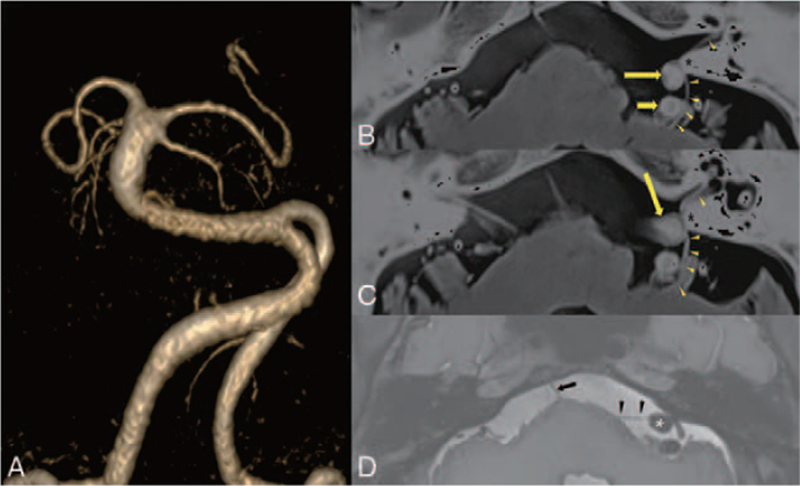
Magnetic resonance imaging (MRI) and angiography (MRA). 3D time-of-flight (TOF) MRA shows laterally displaced course of the distal segments of the intracranial vertebral arteries and mid to proximal segment basilar artery (A). Fusion image of proton density MR cisternography and TOF-MRA shows distal cisternal segment of the left facial nerve (arrowheads in B) and the left vestibulocochlear nerve (arrowheads in C) are jammed in between the laterally deviated dolichoectatic right vertebral artery (long arrow) and posterior edge of the internal acoustic meatus (asterisks). The proximal cisternal segment of the left facial nerve (arrowheads in B) is stretched by the left vertebral artery (short arrow). Axial proton density MR cisternography (D) demonstrates a laterally deviated course of the left abducens nerve (arrowheads) by the right vertebral artery (asterisk) compared to a normal course of the right abducens nerve (arrow) entering Dorello canal.
